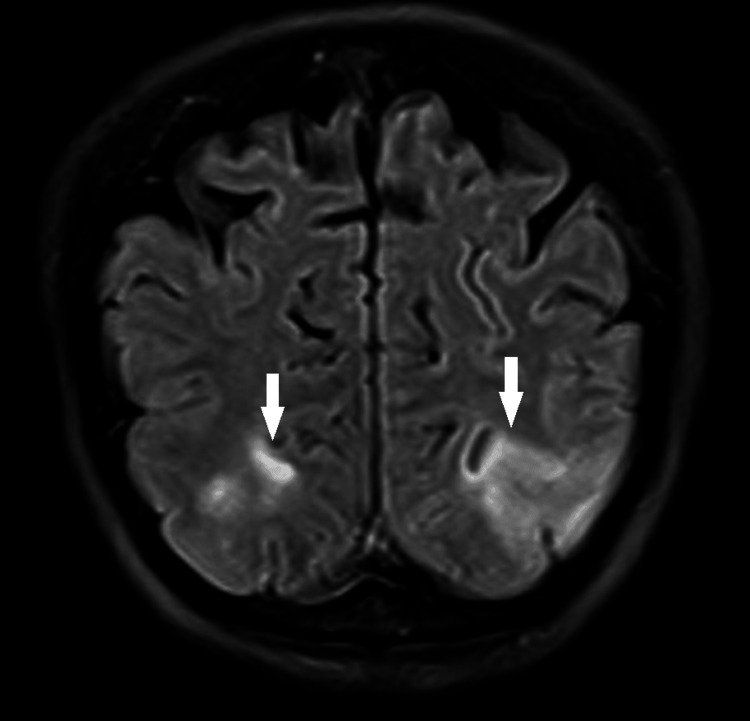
Coronal plane of brain magnetic resonance imaging (MRI) showing an increased bilateral signal intensity involving the cortex and subcortical white matter of the occipital lobe (Arrows) on the T2-weighted fluid-attenuated inversion recovery (FLAIR).
